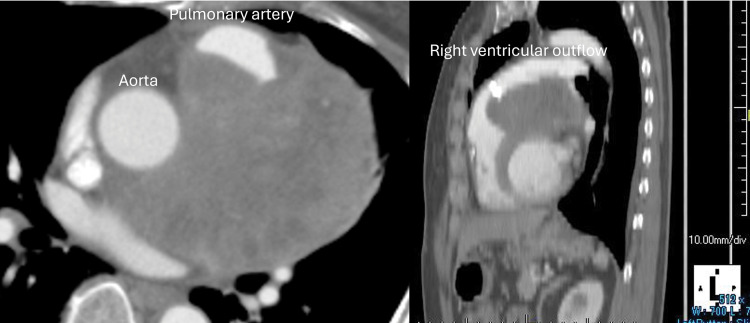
At 4 months after surgery, computed tomography showed progression of mediastinal tumor.
